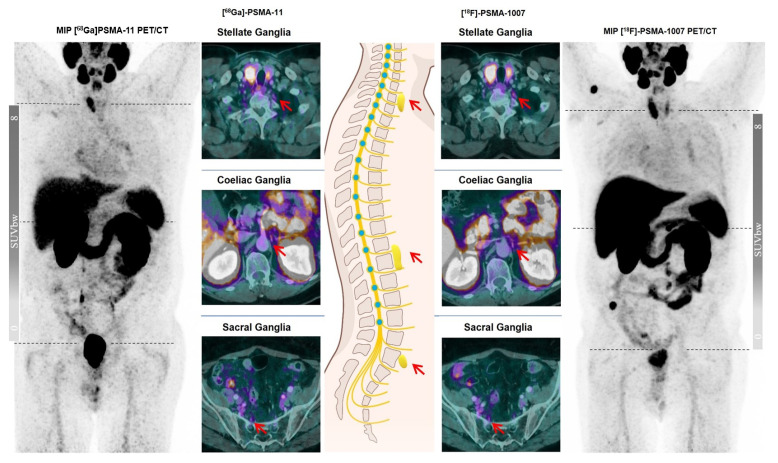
A 79-year-old male patient in follow-up after biochemical recurrence of prostate cancer with varying visual detectability of ganglia at the typical sites (red arrows) on different PSMA ligands.
